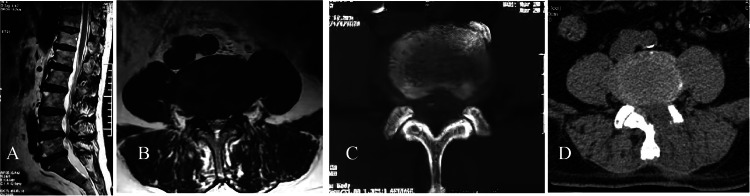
Case 16, a male patient aged 73 years, with lower back pain, left lower extremity pain and intermittent claudication for 5 years. (A‒B) Preoperative MRI showed lumbar spinal stenosis in L_4,5_ space and LIDH. (C) After the first surgery, CT showed that the herniated intervertebral disc tissue compressed the nerve. (D) After MMED, CT showed adequate decompression.
